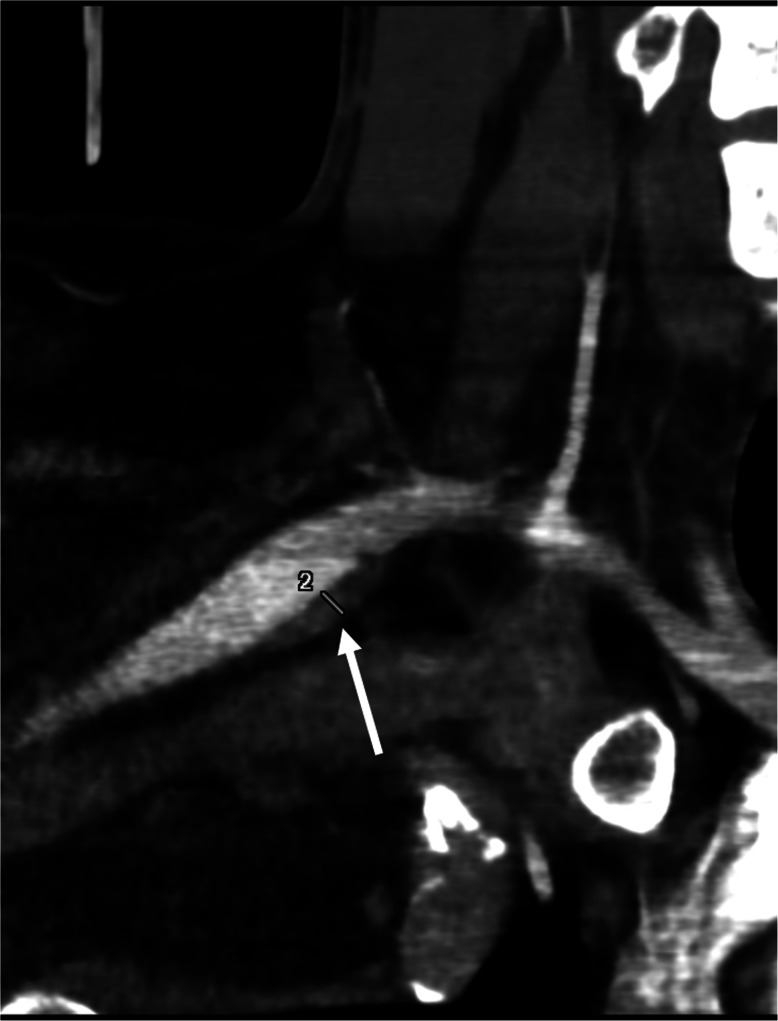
CT angiography showing a 24.1 mm thrombus (arrow) in the subclavian artery
